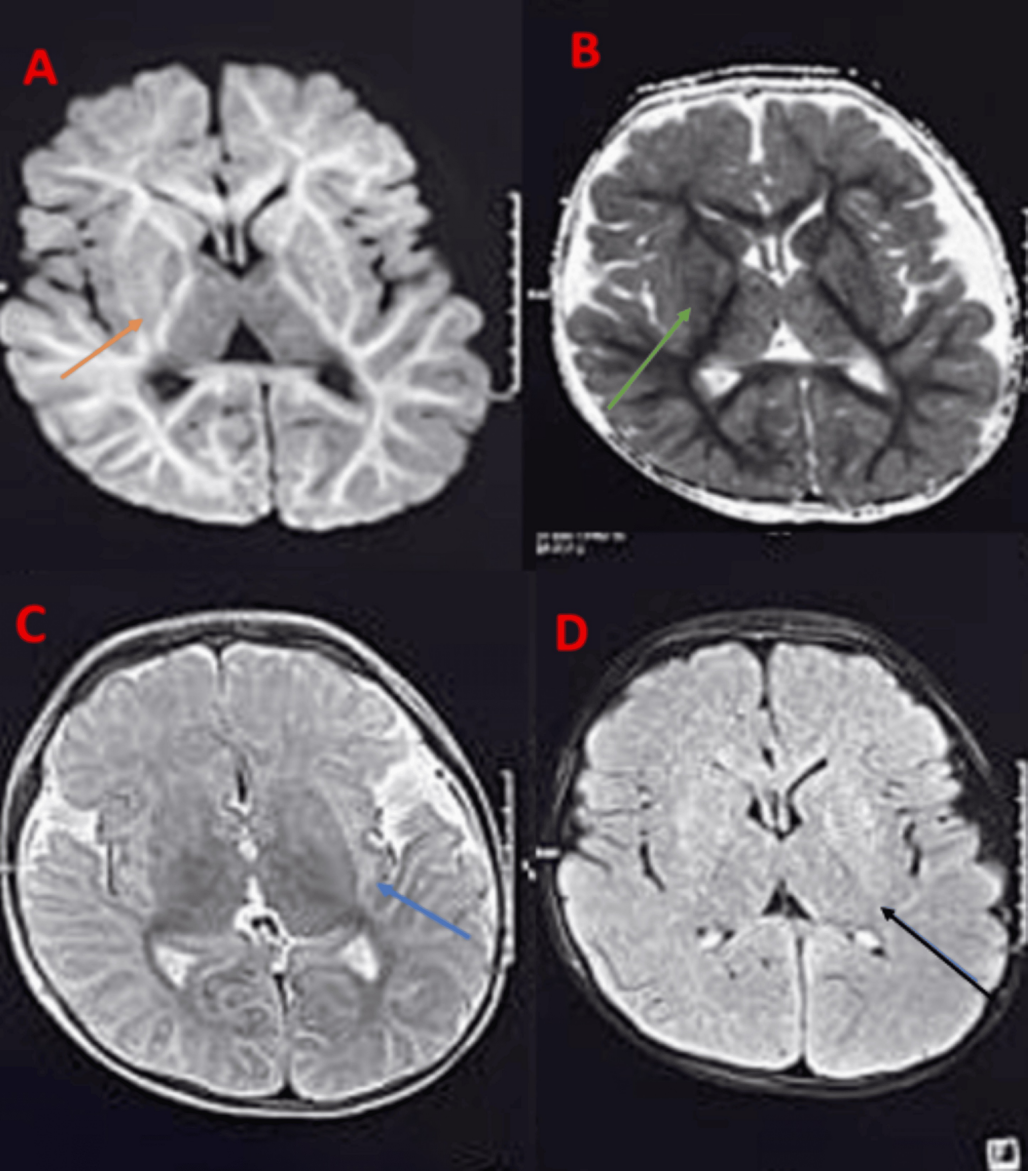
MRI brain A: diffusion-weighted imaging (orange arrow). B: apparent diffusion coefficient (green arrow), showing diffusion restriction involving the entire subcortical white matter, corpus callosum, centrum semiovale, internal and external capsule. C: T2 axial section, showing external capsule hyperintensity (blue arrow). D: FLAIR axial section, showing hyperintensities in the corpus callosum and internal and external capsule (black arrow)
